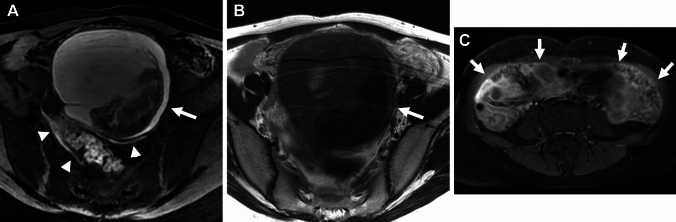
Ruptured endometrioma. A 26-year-old with acute abdomen. MRI is obtained at 3 days after the onset. A Fat-saturated T1-weighted image and B T2-weighted image show a left endometrioma (arrow) with a lack of tension. High signal intensity peritoneal fluid collection (arrowheads) is revealed on fat-saturated T1-weighted image. Diffuse intense contrast enhancement (arrows) is observed on C the post-contrast fat-saturated T1-weighted image reflecting chemical peritonitis
